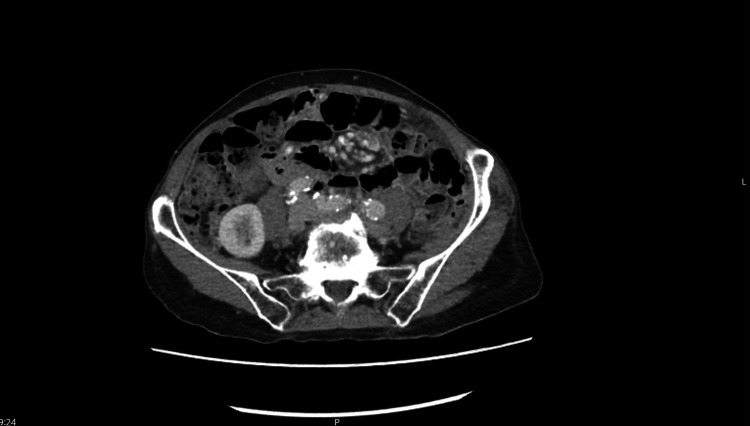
CT abdomen and pelvis with IV contrast showing mildly enlarged anterior paracardiac lymph nodes, progressive ascites, and multiple newly developed left-sided omental nodules, consistent with evolving peritoneal disease.
